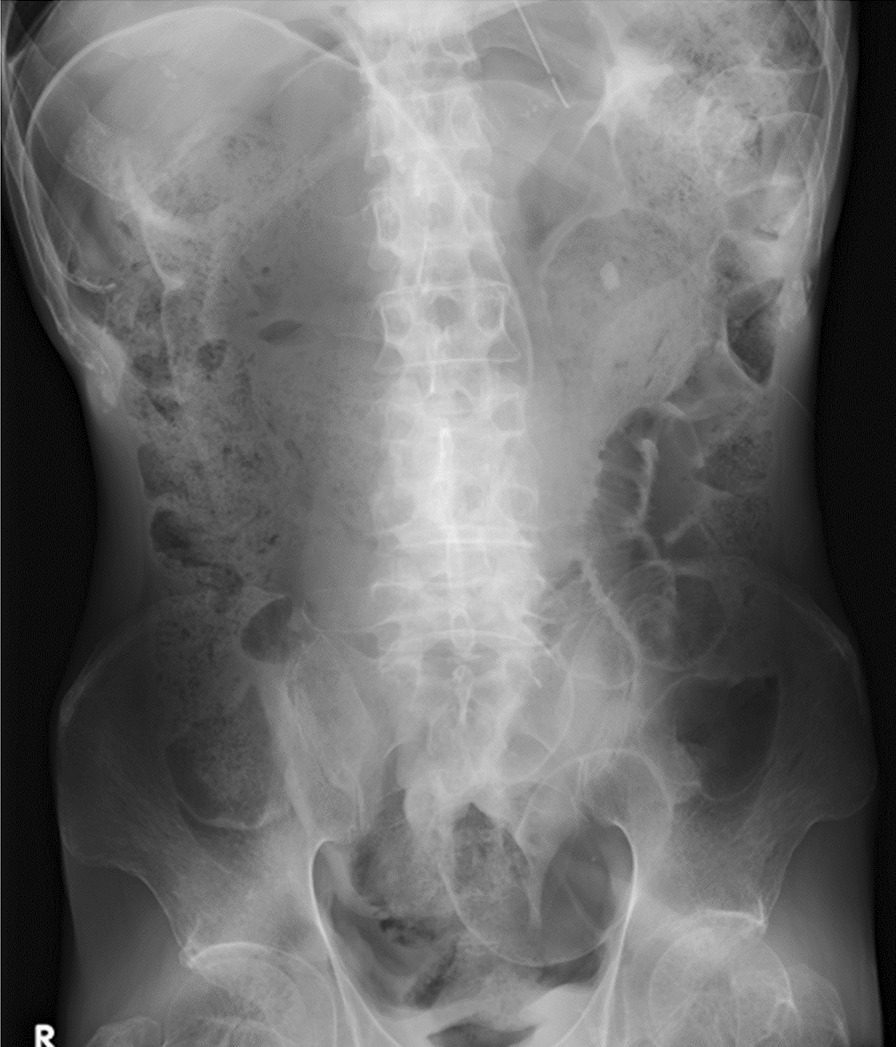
An abdominal X-ray on the 41st hospital day revealed relief of dilated sigmoid colon and rectum together with massive amount of stool
